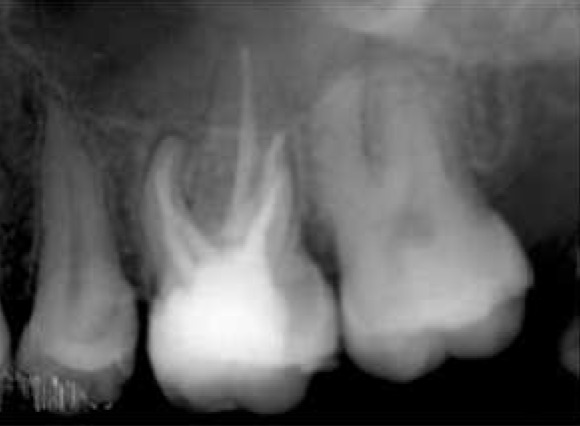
The final radiograph of tooth #26
